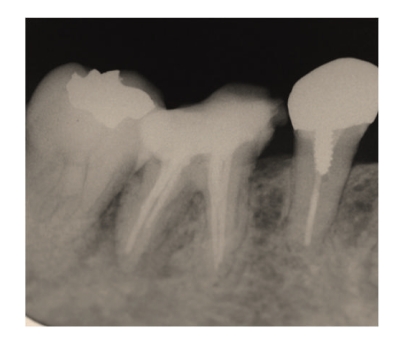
Case 2: Radiography at the root canal filling showing remaining bone loss at the root apex.
